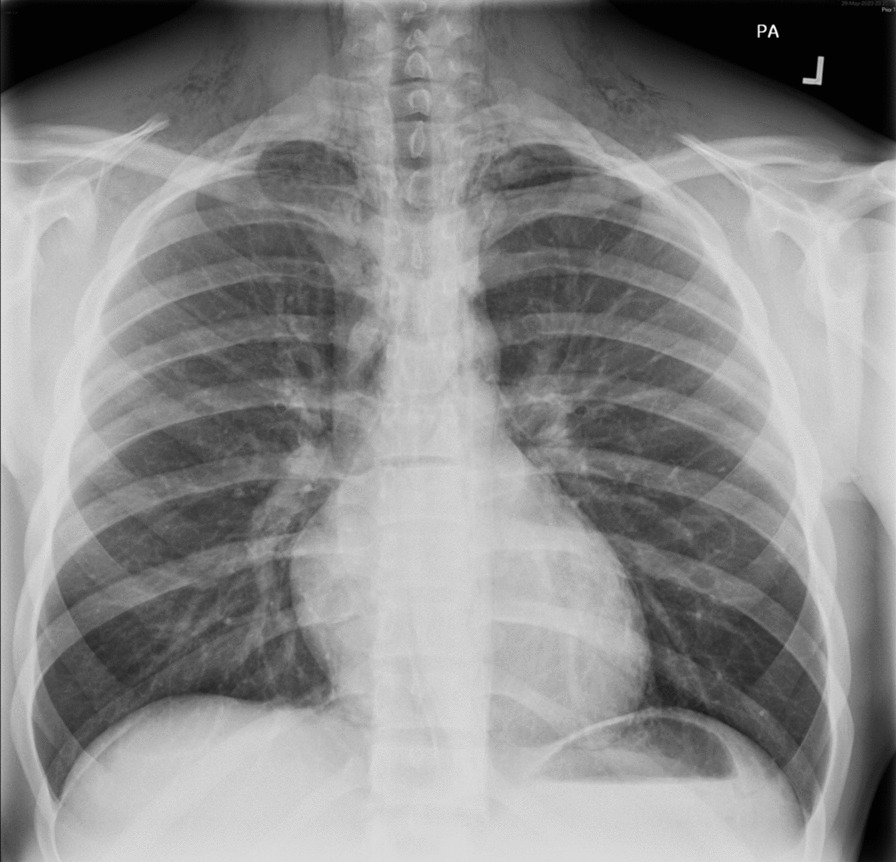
Posteroanterior chest radiograph demonstrating widespread surgical emphysema over the superior thorax and neck bilaterally
